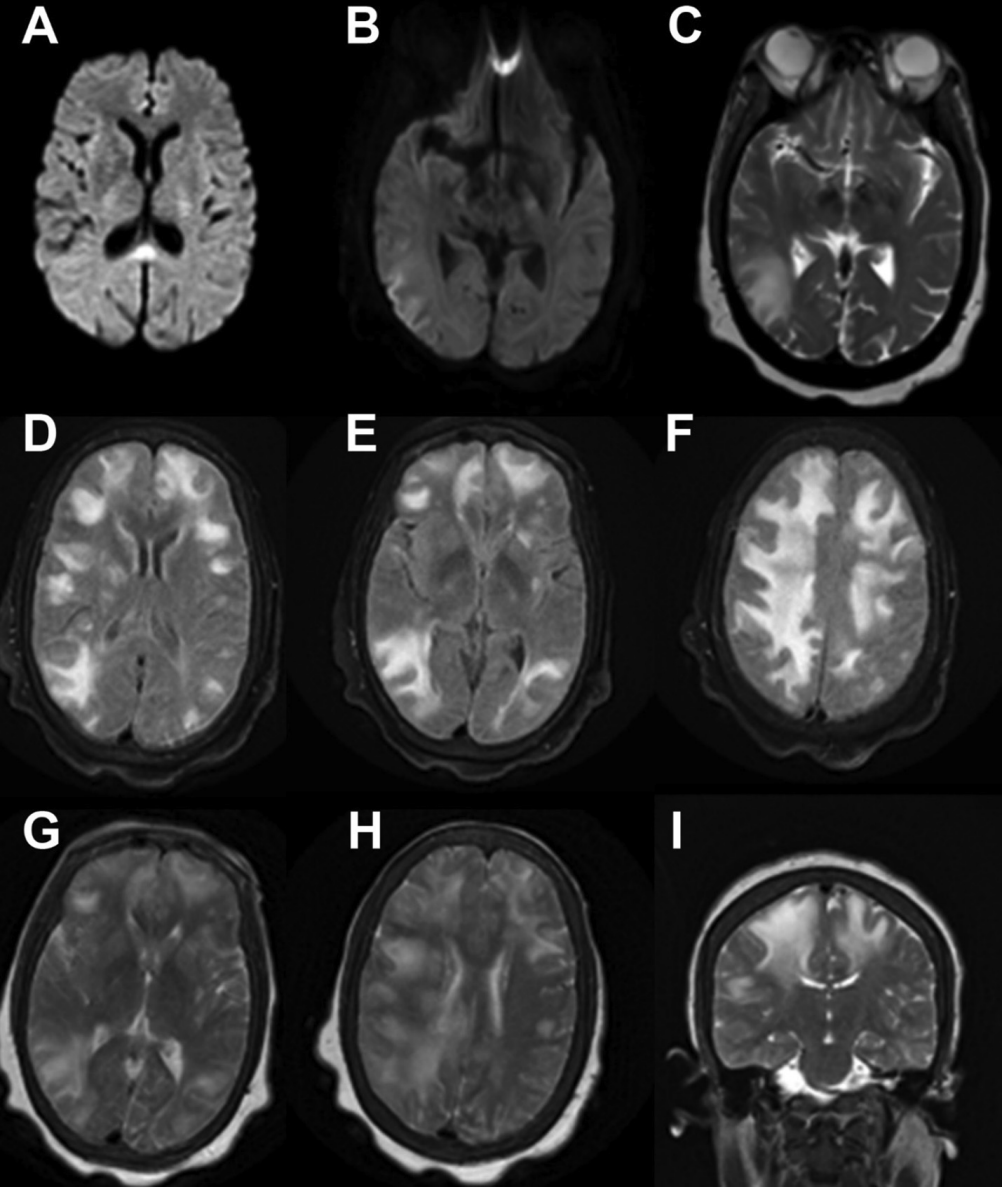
First brain MRI DWI (A) with cytotoxic edema in the splenium of the corpus callosum along with a possible small area of mild cytotoxic edema in the left cerebral white matter Supplementary MRI sequence A. Second brain MRI DWI (B), Axial T2 (C) and Axial Flair (D). Third brain MRI, axial FLAIR hyperintensities (E- I). Supplementary MRI sequence E-I.
